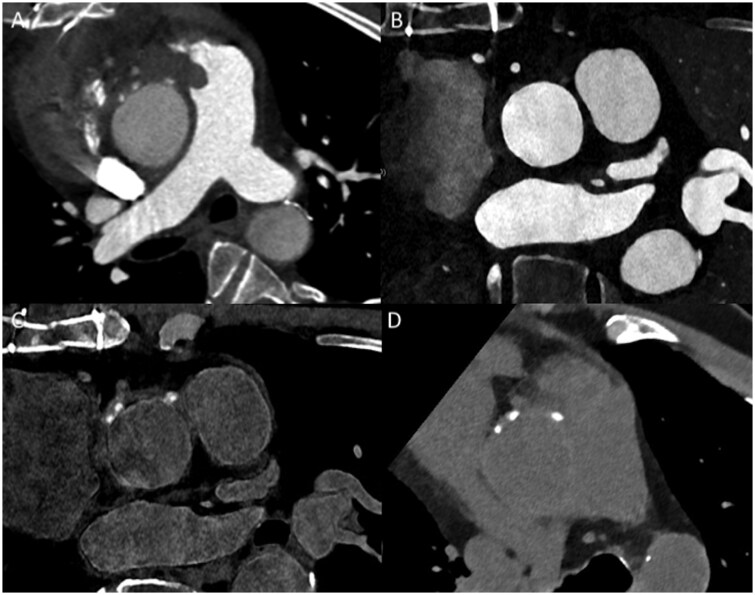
Material decomposition maps in a patient who presented 3 weeks post aortic root abscess debridement patch repair. The abnormality on CTPA (Panel A) was further evaluated on PCD-CT using an iodine only image (Panel B) and a virtual non-contrast image (Panel C) to demonstrate intramural calcification/surgical clips. This was confirmed with a true non-contrast image (Panel D).
